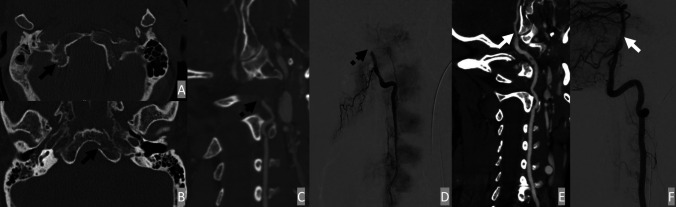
Vertebral arteries dissection from occipital condyle fractures. A 53-year-old male presented with bilateral occipital condyle fractures after MVC (A and B). The right vertebral artery curve reformat from the initial CTA neck (C) demonstrates abrupt tapering with the absence of contrast opacification of the V3 and V4 segments, compatible with flow-limiting dissection. The right vertebral artery injection of DSA (D) demonstrated occlusion of the V3 segment. The left vertebral artery curve reformat from the CTA neck (E) showed vessel irregularity of the left V4 segment. Left vertebral artery injection of DSA (F) demonstrated a short segment of non-flow limiting dissection
